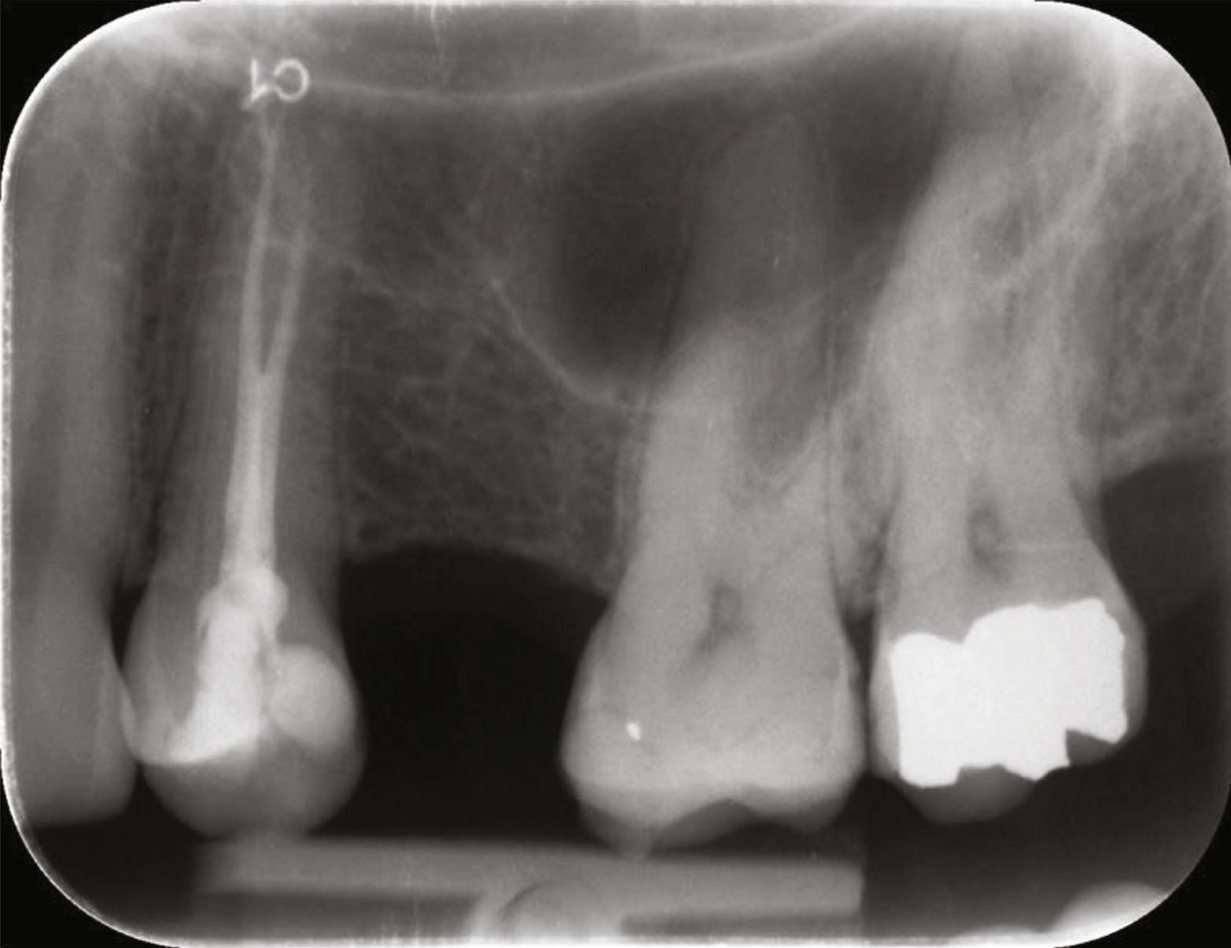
Preoperatory endoral radiography.
